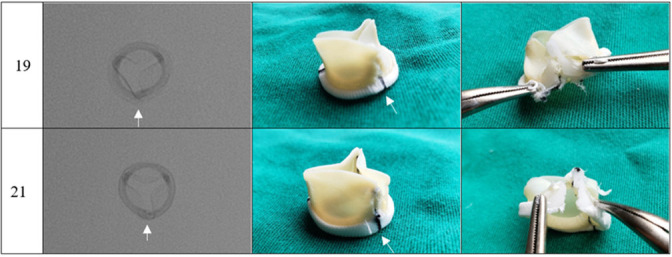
Labcor Dokimos prosthesis after fracture, sizes 19 mm and 21 mm. Radiography (superior-inferior projection) and photographs (intact lining and dissected lining). Arrow corresponding to the fracture site.
